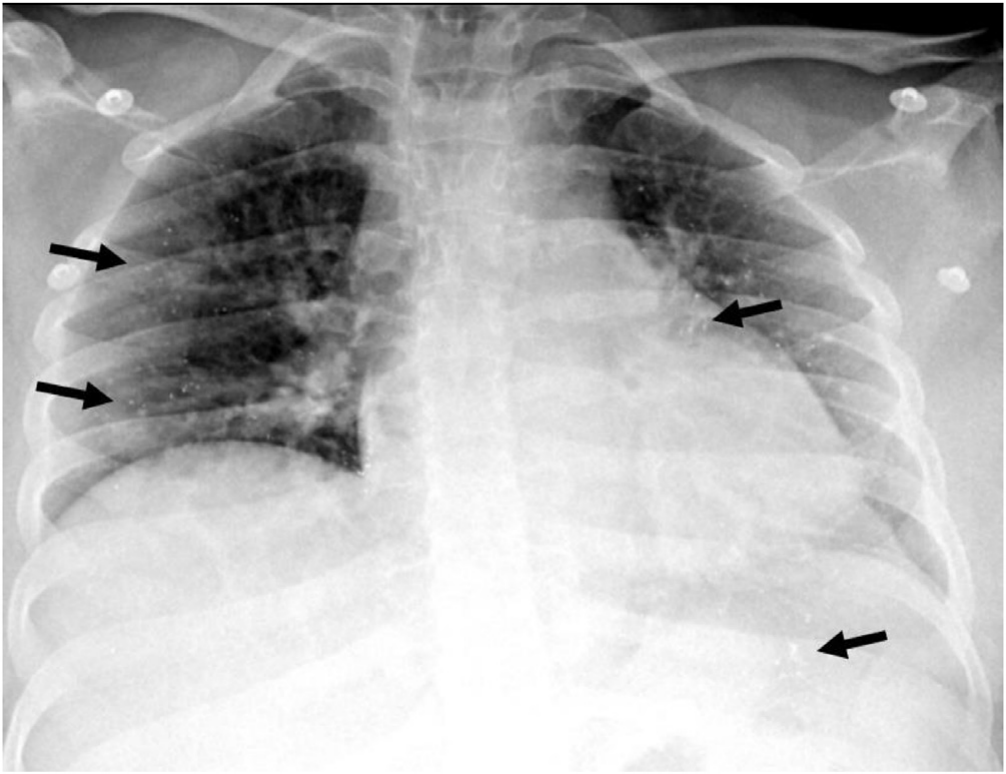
Chest radiography, AP view. There are some radiopaque nodules in the lung parenchyma. These nodules are closely related to the pulmonary vasculature.
